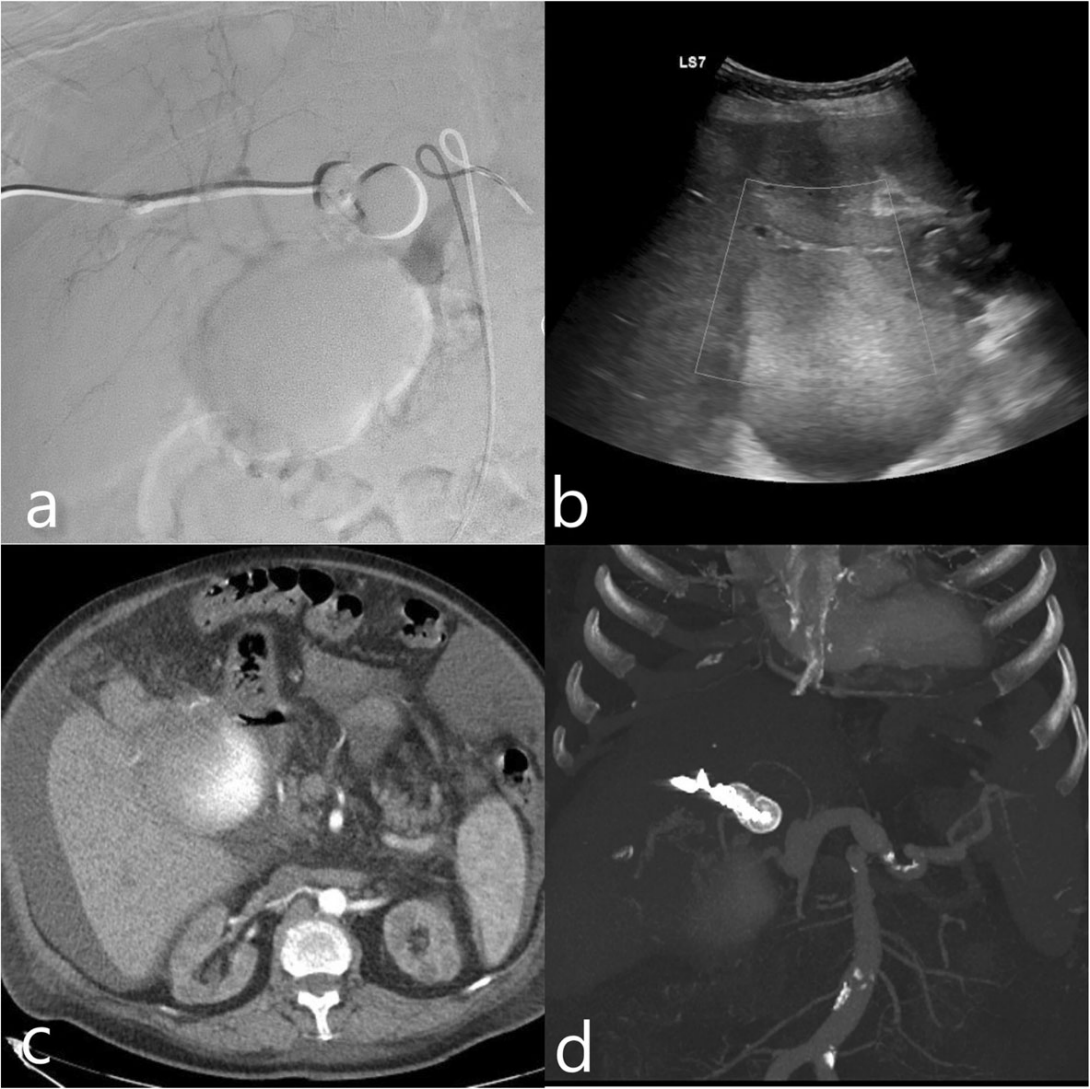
Celiac angiogram and US images (a-b) showing immediate thrombosis formation in the portal vein and aneurysm sac while preserving hepatic artery circulation. One month follow up triphasic CT(c-d); arterial phase shows no filling of the portal vein and aneurysm sac following the AVP II; coils deployed through the access site can be seen
